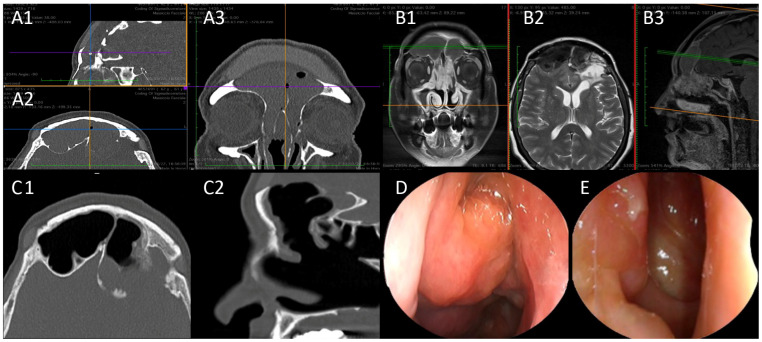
A 62-year-old woman with NSAID-ERD and medical history of multiple brief cycles of OCS in the last few years (>52 cumulative days/year). The patient had recalcitrant frontal sinusitis associated with invalidating frontal pain. Two previous surgeries including an external bicoronal approach to the frontal sinus and reconstruction with custom-made prosthesis. Diagnosis of recurrence of nasal polyps and mucocele was made in January 2023. After 12 months of dupilumab, the mucocele and nasal polyps had resolved. Herein we report the results after 12 months of treatment. (A1–A3) Pre-treatment CT-SCAN, complete opacification of the frontal sinuses ((A1) Sagittal view. (A2) Axial view. (A3) Coronal view). (B1–B3) Pre-treatment MRI showing recurrence of the nasal polyposis and frontal mucocele ((B1) Coronal view. (B2) Axial view. (B3) Sagittal view). (C1,C2) Post-treatment CT showing pneumatization of the frontal sinuses ((C1) Axial view. (C2) Sagittal view). (D) Pre-treatment endoscopic view. (E) Post-treatment endoscopic view.
